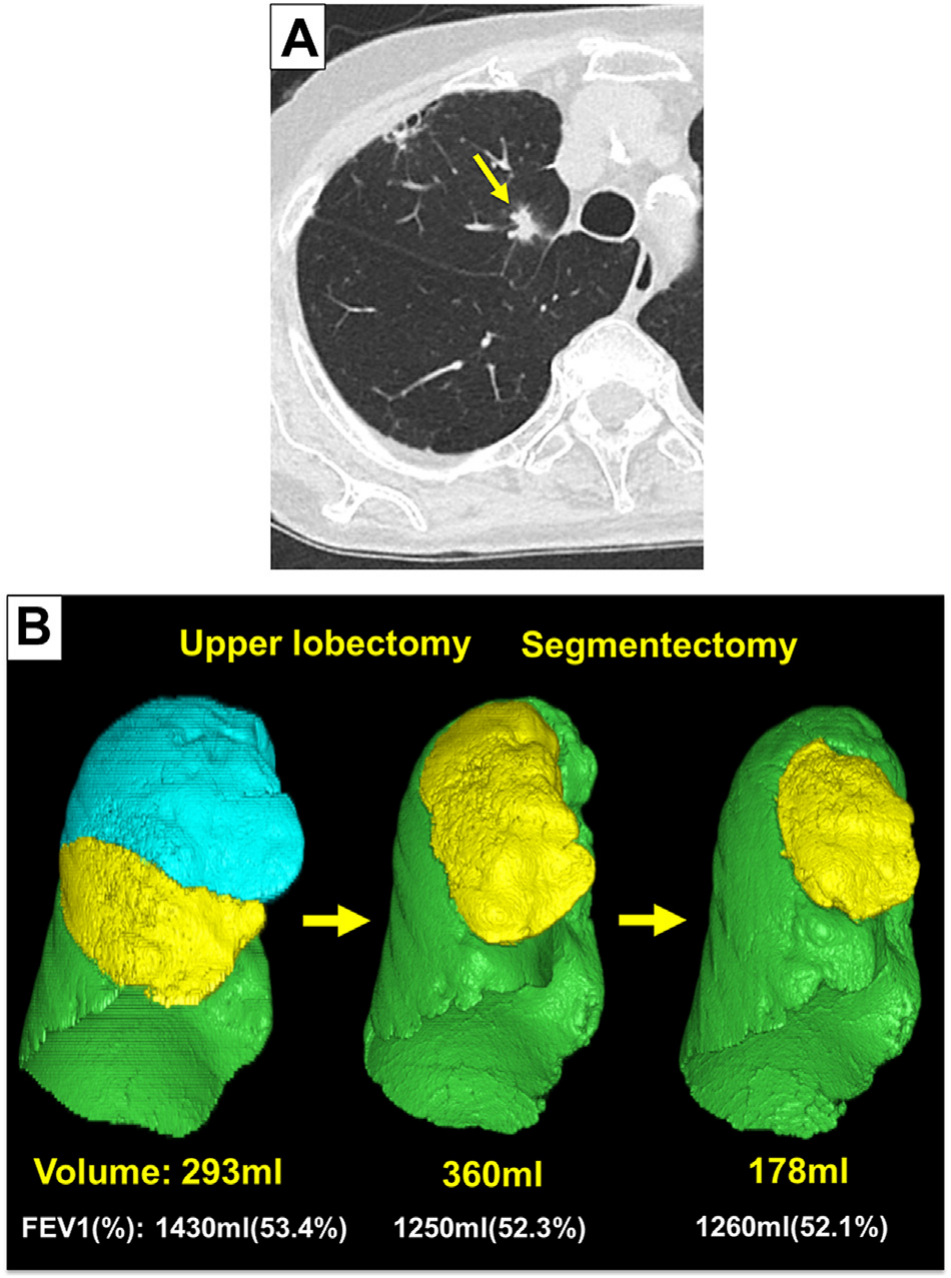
Representative computed tomography (A) and three-dimensional computed tomography images from lateral views before right upper lobectomy, after right upper lobectomy, and after right S4 segmentectomy (B). (A) Yellow arrow showing a 14-mm part solid nodule (solid component: 10 mm) in S4a in right middle lobe. (B) Lower label showing the volume of the right middle lobe and forced expiratory volume in one second (FEV1). Computed tomographic images were transferred and analyzed in three dimensions, and the volume of each lobe was calculated using Synapse Vincent (Fuji Film Co., Ltd., Tokyo, Japan).
